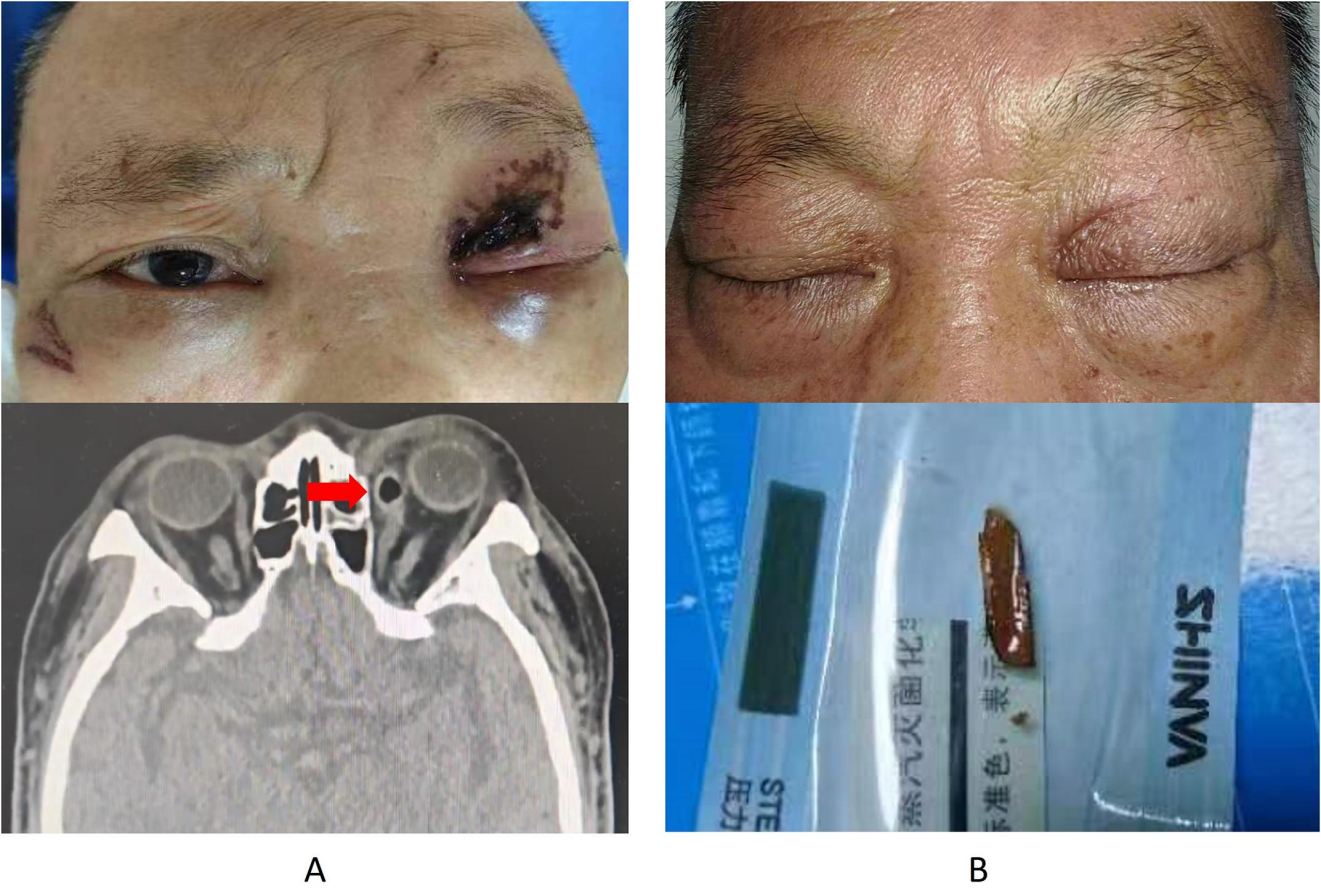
Clinical imaging and photos of first surgery. (A) Photograph showing Left eye exhibited redness and swelling and computed tomograph showing “gas formation”. (B) Photograph of wound healing and wooden fragment of foreign body. Red Arrow: gas formation.
